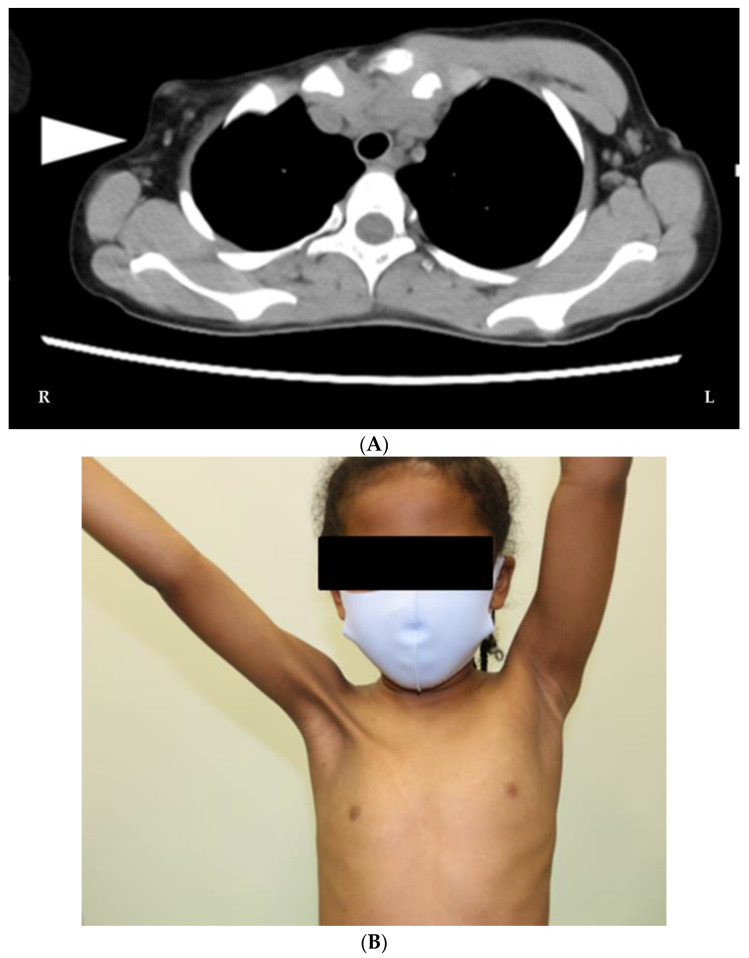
(A) Chest CT scan of a 10-year-old girl with axillary contractures revealed the absence of pectoral muscles in the right thoracic wall (white arrowhead). (B) Preoperative photograph of a 10-year-old girl with PS and axillary contractures demonstrating limited range of motion of the right shoulder joint.
